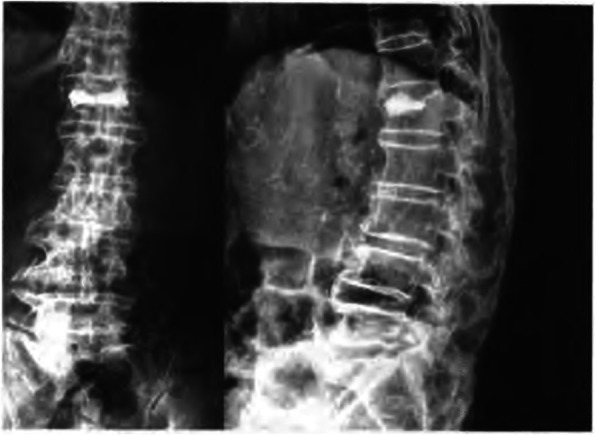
X-ray film after PVP shows a small amount of bone cement in the OVCF
